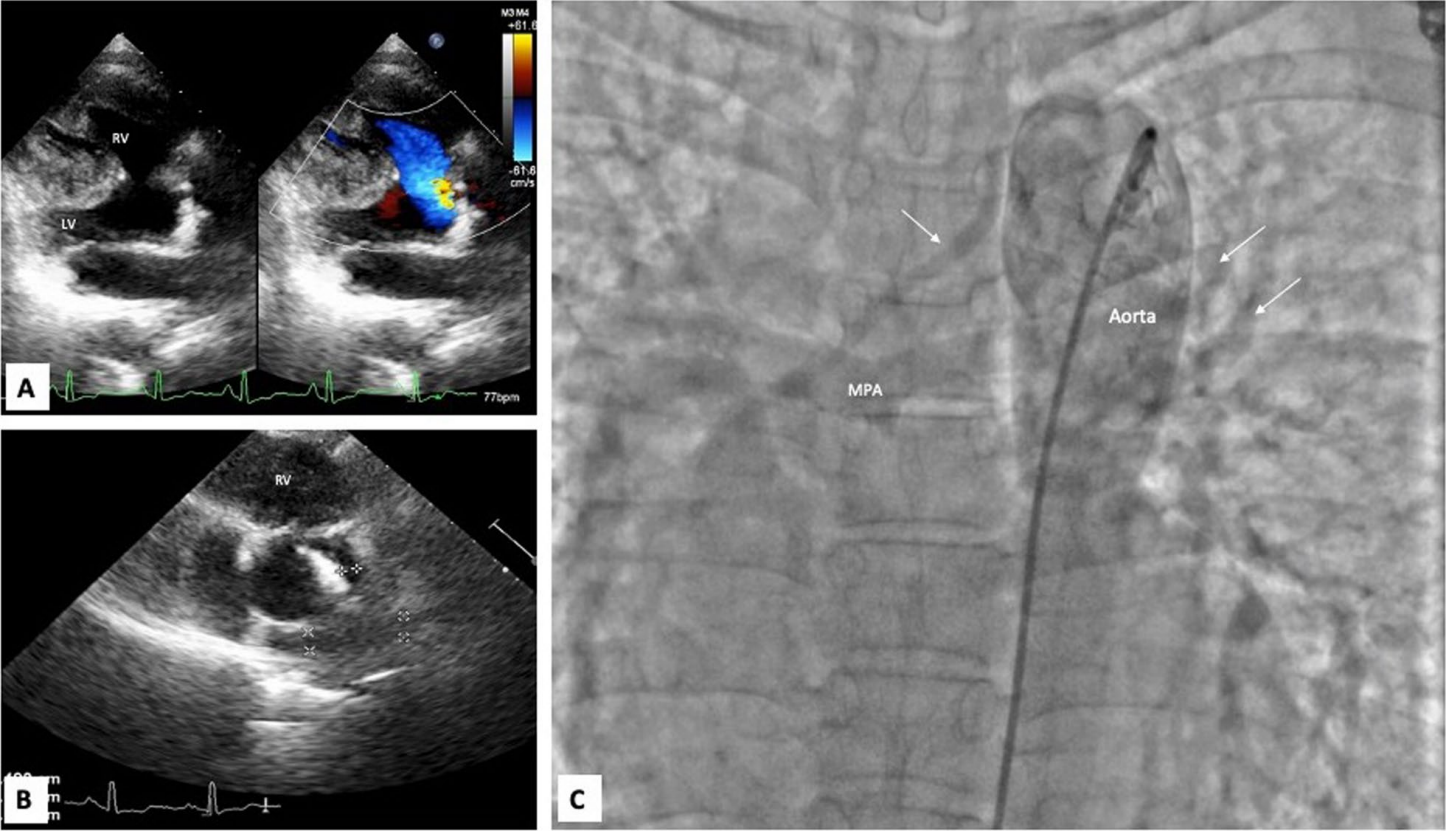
A Parasternal long axis view of echocardiography showing large ventricular septal defect with right to left shunt, right ventricular hypertrophy and malaligned aorta. B Parasternal short axis view of echocardiography at the level of aortic valve showing pulmonary valve atresia and confluent small pulmonary arteries. C Invasive angiogram with pigtail catheter in descending thoracic aorta showing major aortopulmonary collaterals (white arrows) filling the confluent pulmonary arteries
